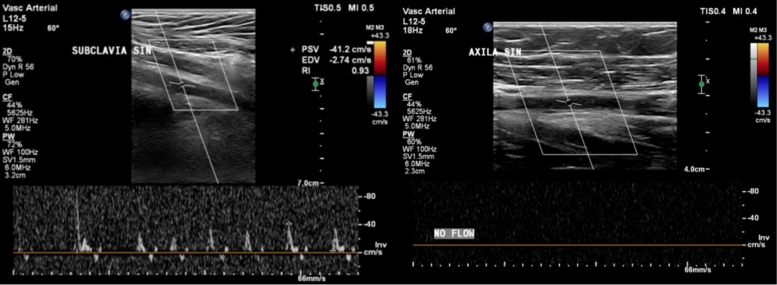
DUS Demonstrating Absence of Flow in Left Axillary Artery Bedside DUS showed an absence of flow in the left axillary artery, confirming arterial occlusion. This finding established the diagnosis of ALI classified as Rutherford grade IIb, indicating an immediate need for revascularization to prevent irreversible tissue damage. ALI = acute limb ischemia; DUS = Doppler ultrasound.
